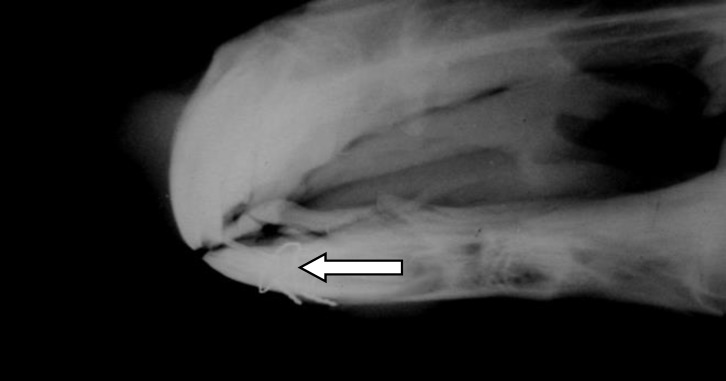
Radiography of  cerclage wire (arrow) for rostral mandibular fracture in a 3-year-old Arabian colt.
